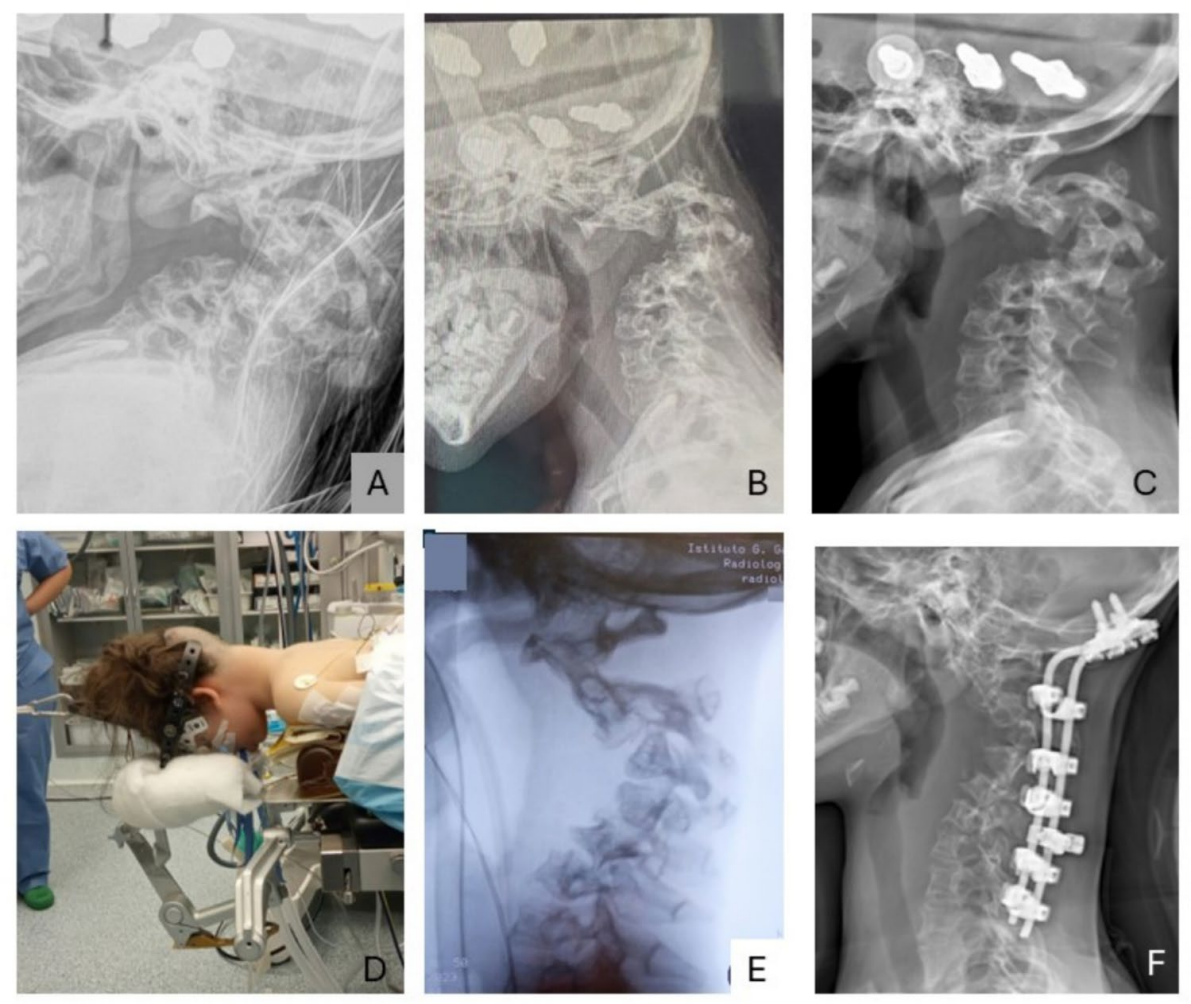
Progressive correction of the deformity. X-ray of the column before traction (A); soon after the traction procedure, an immediate reduction of the kyphosis was achieved (B); 6 weeks after traction (C). The patient is in traction on the surgical bed (D). Further traction under anaesthesia showed further improvement and correction of the kyphosis (E). Post-operative radiological X-ray (F)
